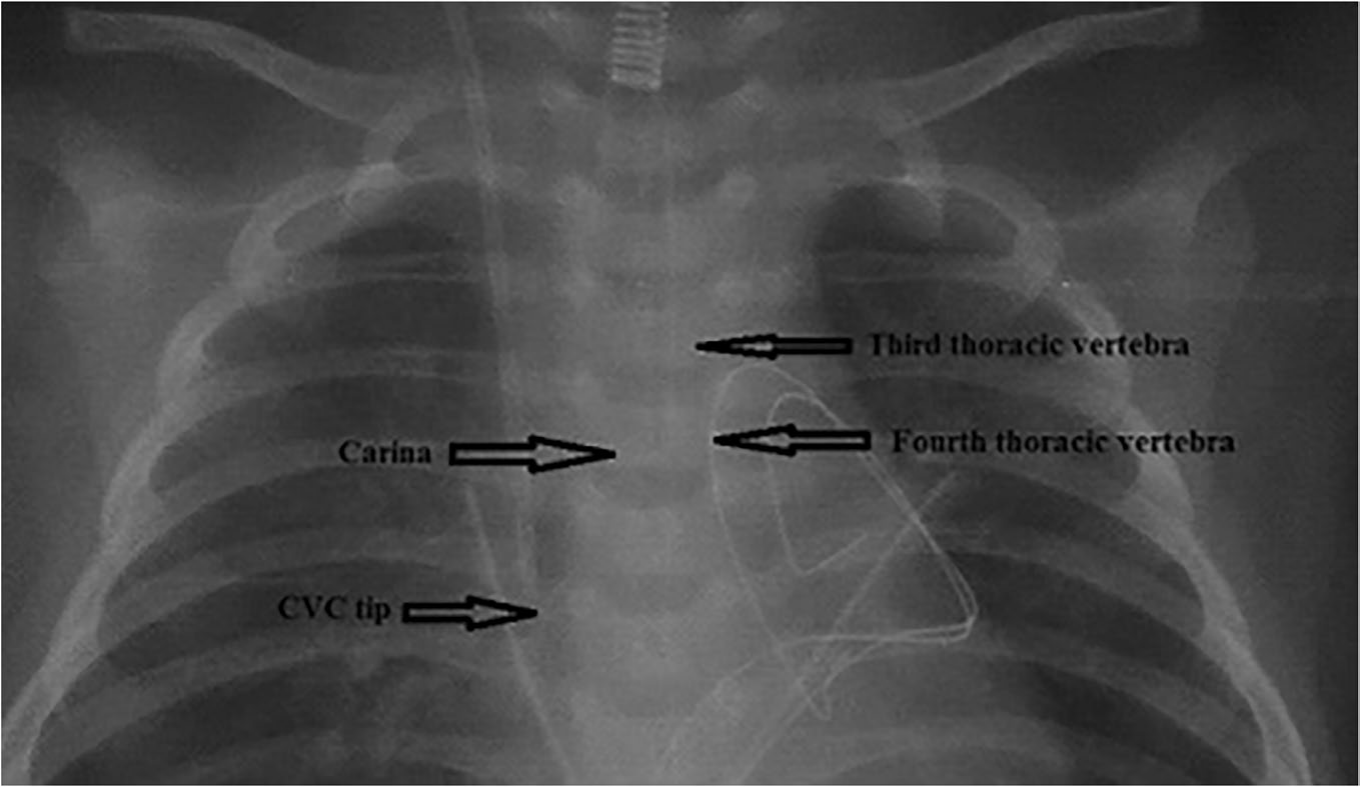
Carina, CVC tip and thoracic vertebra display on the radiograph. *CVC* central venous catheter.
